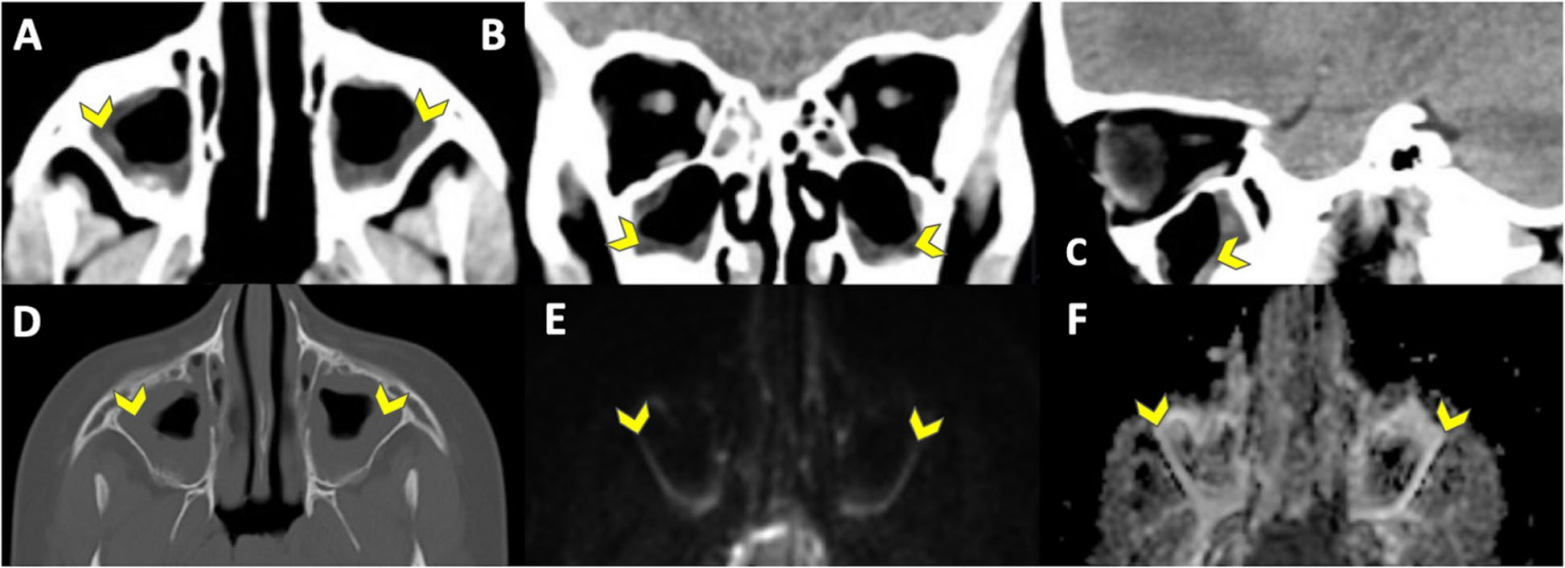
ID14. Paranasal sinus mucosal thickening (yellow head arrow), visible on both EPIMix and CT at the side-by-side consensus. Axial (A), coronal (B), sagittal (C) soft tissue window CT images, axial (D) bone window CT image, and DWI (E), ADC (F) EPIMIX images
